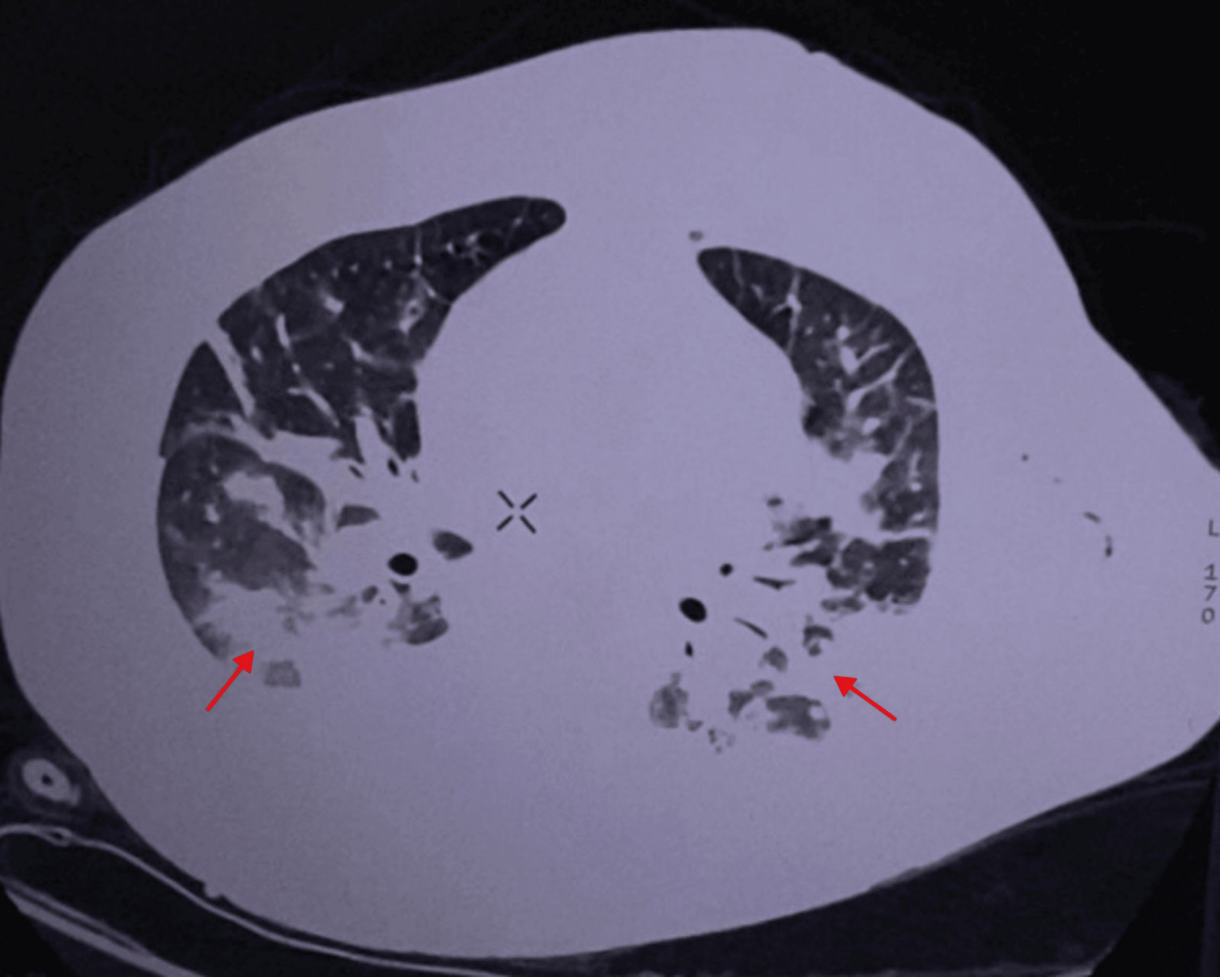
CT scan showing ground-glass opacities and subsegmental atelectasis.
